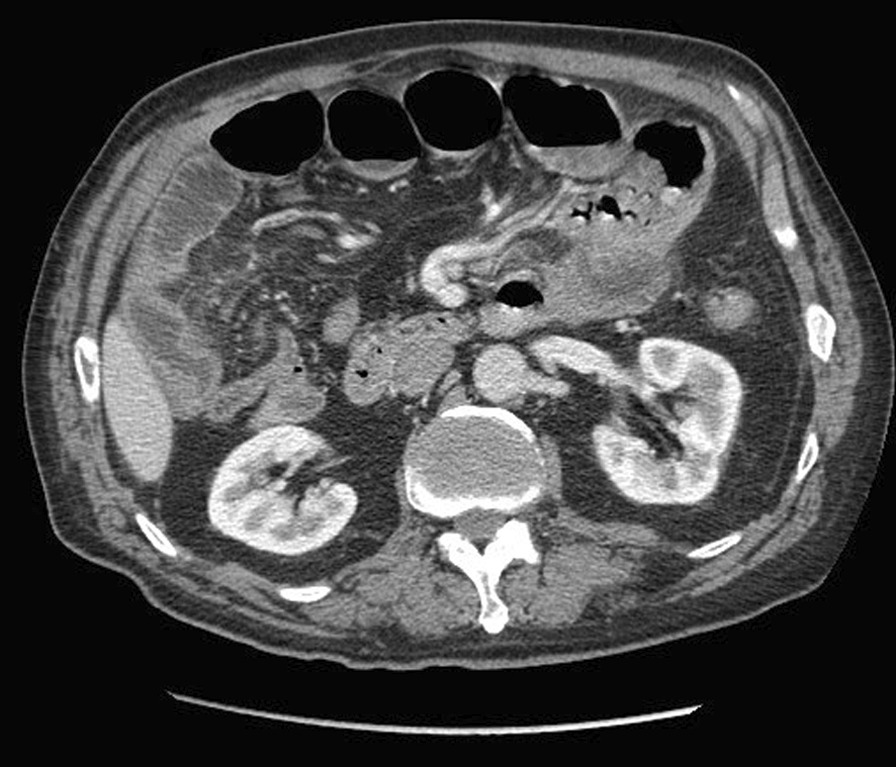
Computed tomography (CT) scan showed air-fluid levels and signs of mechanical ileus
